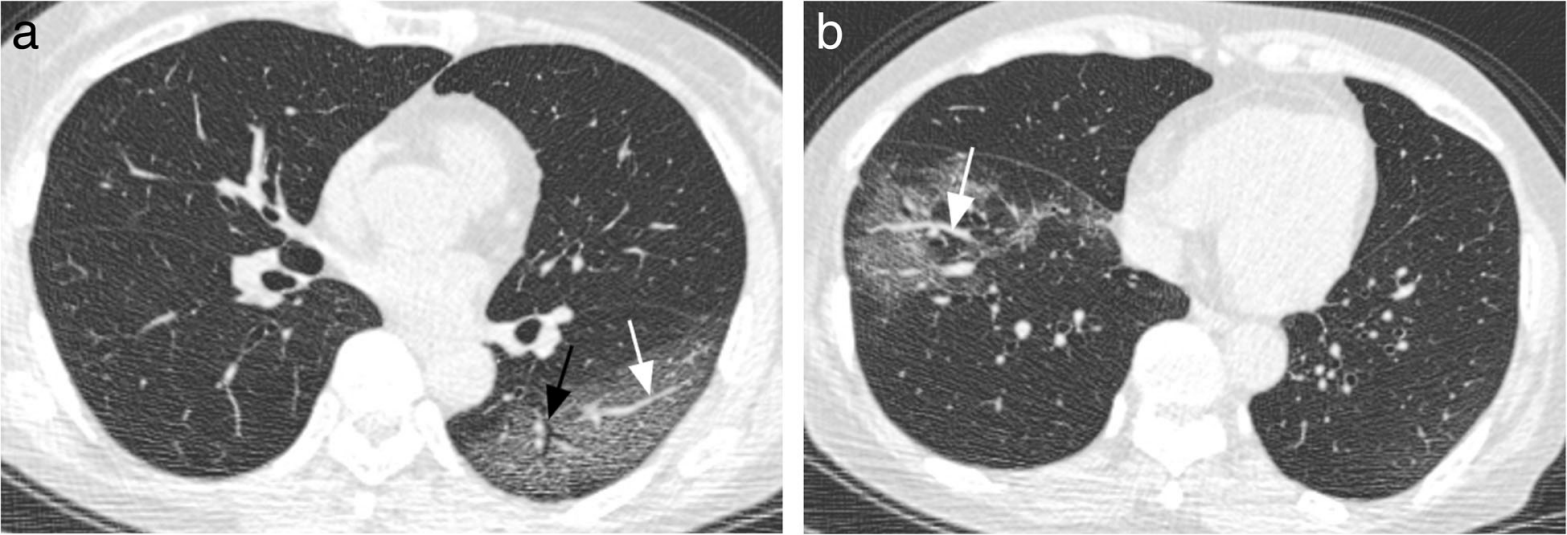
Chest CT images of a patient with mild COVID-19. a Axial CT images showed ground-glass opacity (GGO) in the left lower lobe. Vascular bundle thickening (white arrow), air bronchogram sign (black arrow) and crazy-paving sign were observed in the GGO. b Vascular bundle thickening (white arrow) was noted in the GGO of the right lower lobe
